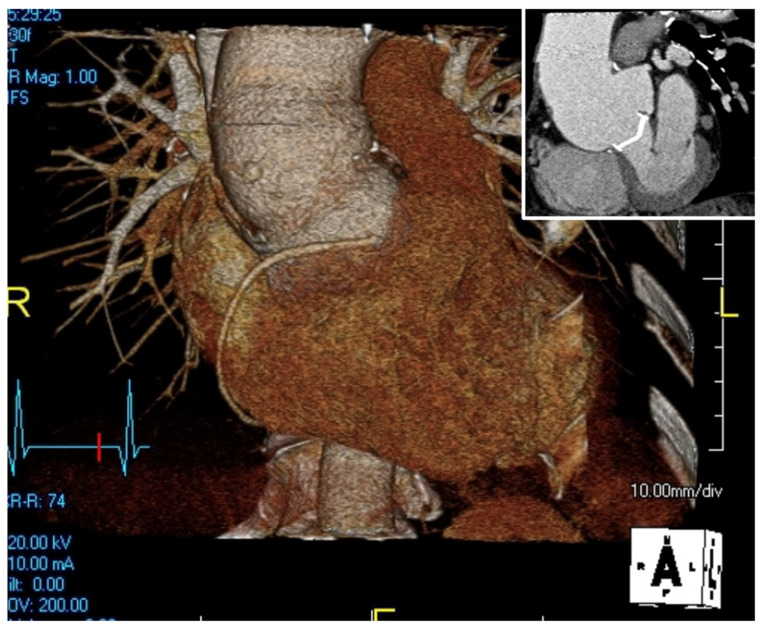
Aortic root aneurysm above the aortic valve prosthesis in 3D reconstruction of the CT scan. The previously implanted mechanical aortic valve can be appreciated in the top corner image—coronal view.
